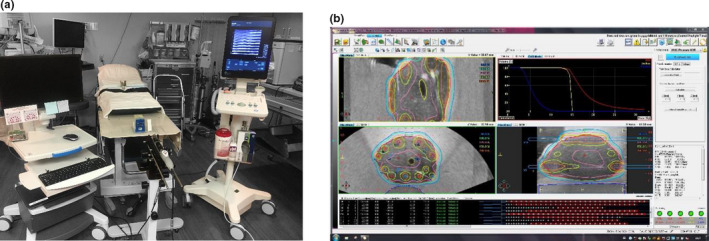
(a) The real‐time ultrasound (US) guided treatment planning system (OncentraProstate) that consists of the treatmenplanning system cart (left), the stepper and stabilizer holding the transrectal US probe (center), and the US unit (right). (b) An initial treatment planned on the TPS (OncentraProstate)
